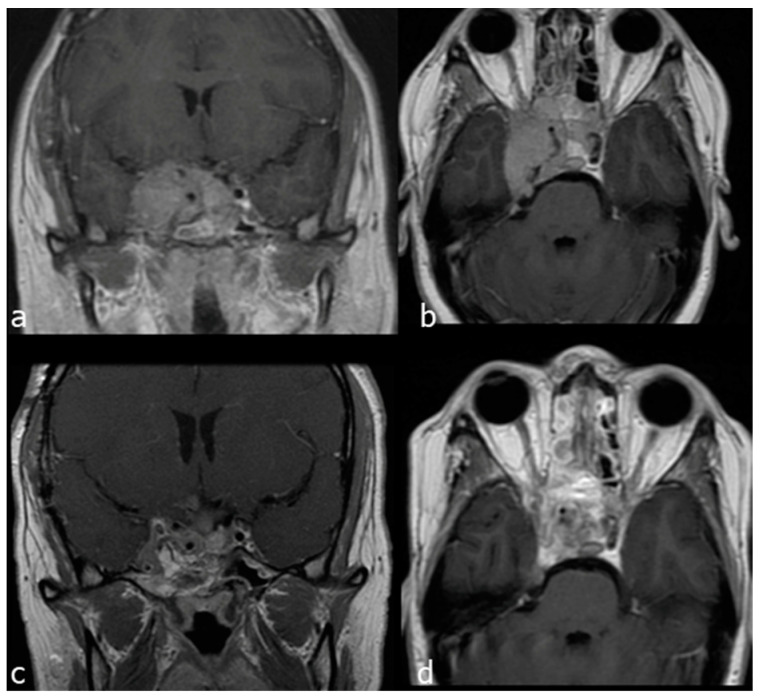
Case 2: preoperative and postoperative MRI scans of a recurrent giant non-secreting pituitary adenoma, previously operated through transcranial and then operated on by a combined EETC and CTM approach. (a) preoperative MRI scan, axial view. (b) preoperative MRI scan coronal view. (c) postoperative MRI scan, axial view. (d) postoperative MRI scan, coronal view.
